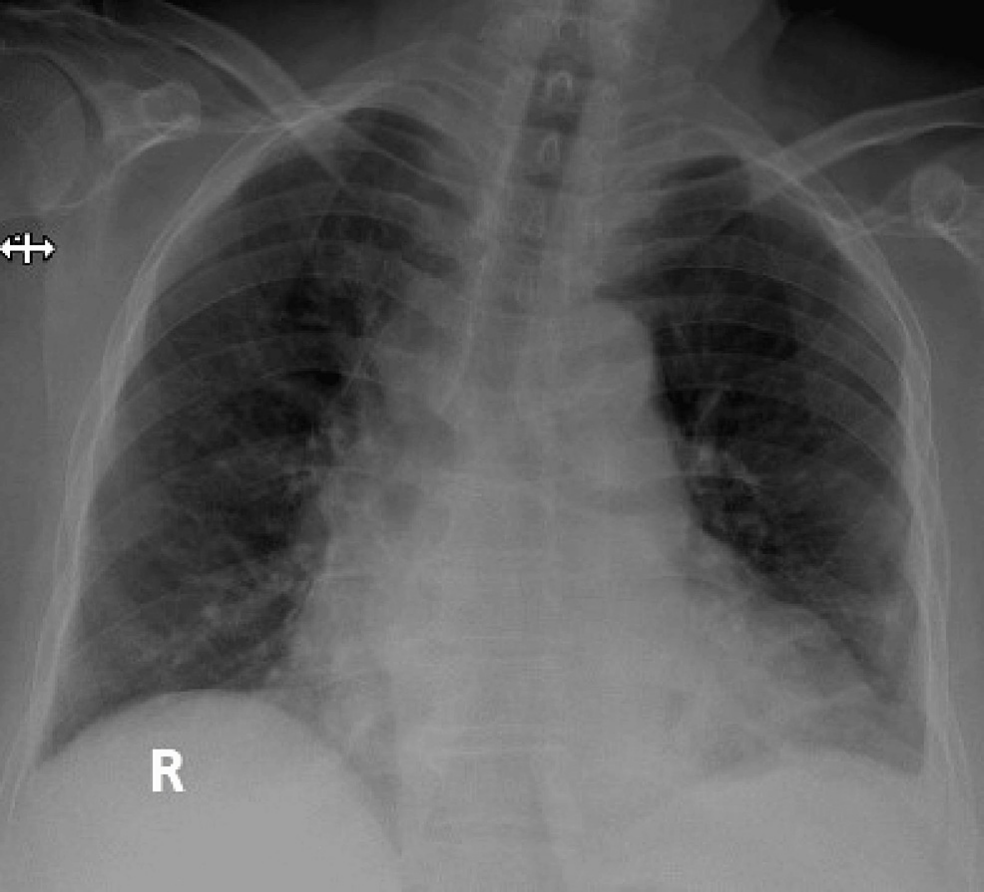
The chest X-ray shows patchy bilateral infiltrations located mainly in the lower and lateral zones.
